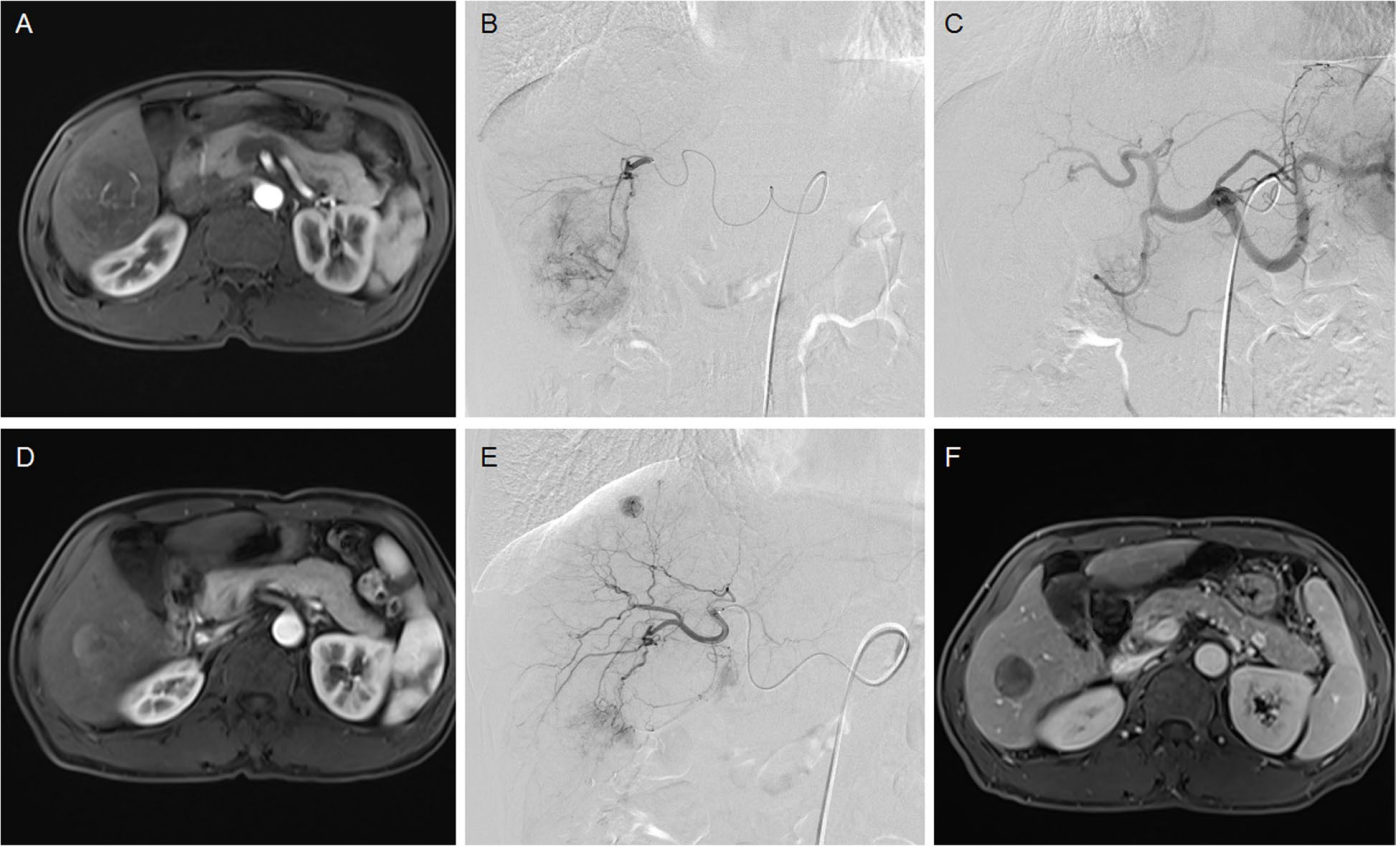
A 53-year male treated by DEB-TACE for advanced primary HCC. A MRI preoperative examination revealed a large tumor of 61.2*48.5 mm in right liver. B-C The tumor artery was superselectively incubated and embolized by 300-500 μm raltitrexed-loaded beads. D MRI revealed a shrunk tumor 1.2 months later. E A small tumor was found and embolized. F The tumor was found to shrink after 8.0 months' follow-up, and still alive after 34.0 months
